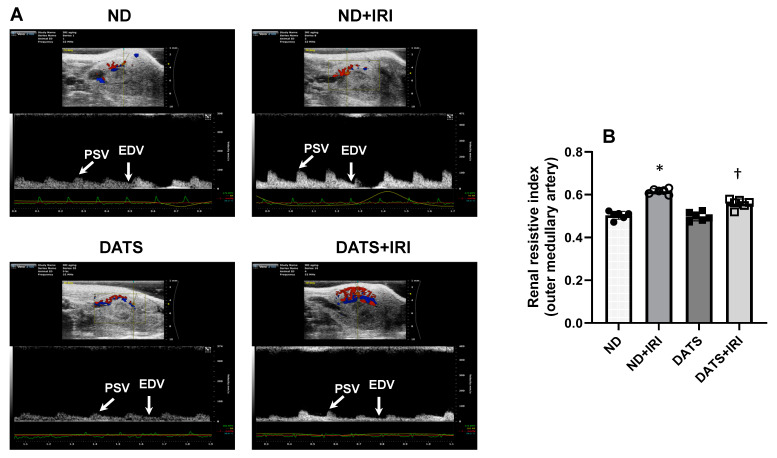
DATS treatment reduced the increased resistive index (RI) of outer medullary arteries caused by IRI in the aged kidney. (A) Representative ultrasound images of the kidney showing color Doppler of vessels in the outer cortico-medullary area. Pulsed-wave Doppler was placed on the outer medullary arteries to acquire data. The resistive index (RI) was measured using the formula RI = (PSV − EDV)/PSV. RI—resistive index, PSV—peak systolic velocity, EDV—end-diastolic velocity. (B) Values are mean ± SD, n = 6/group, * *p* < 0.01 vs. ND, ^†^ *p* < 0.01 vs. ND+IRI.
